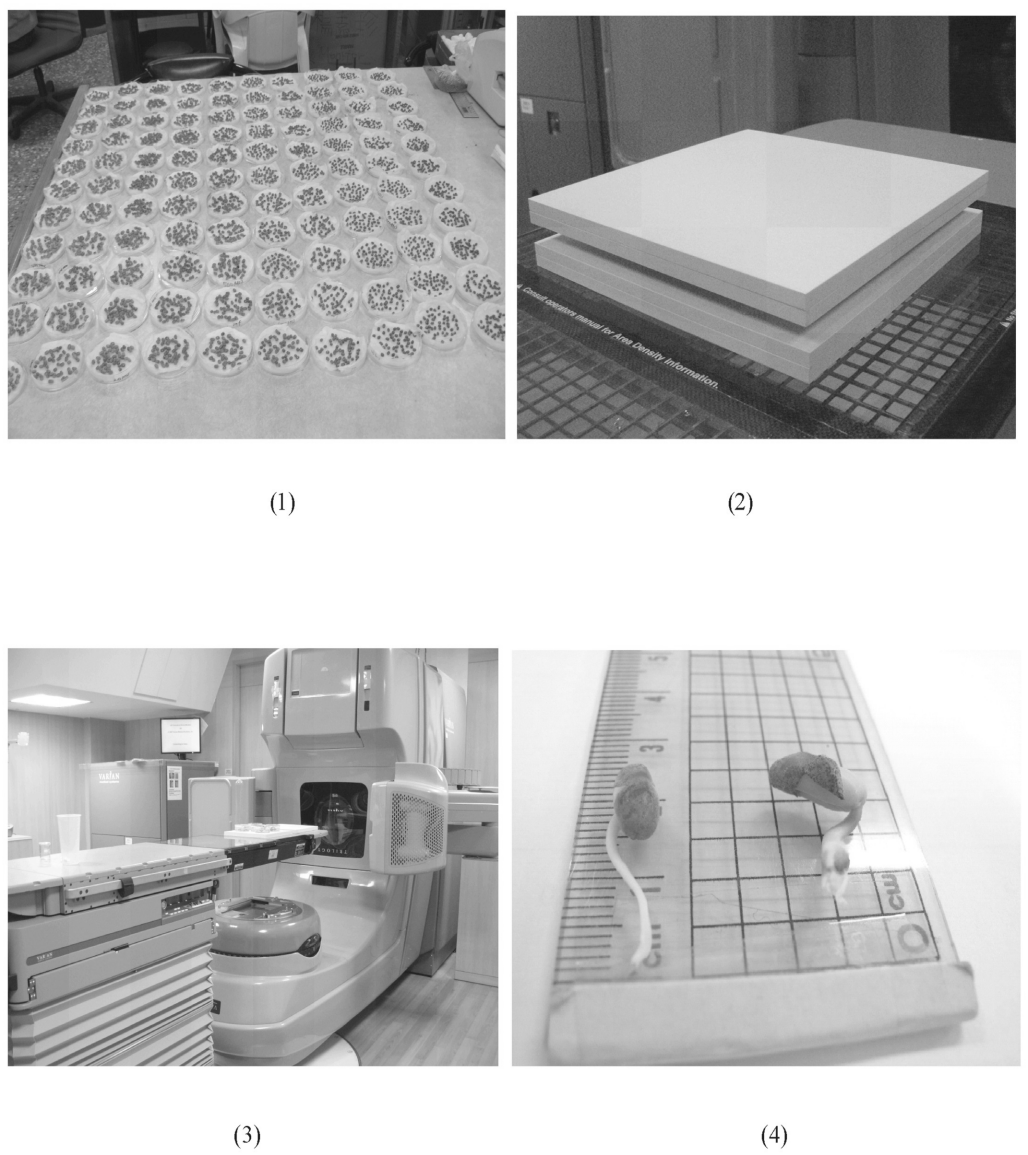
The real situation of mung bean inside the petri dishes (1) during watering, (2) between two 20 mm-thick solid polystyrene plates, (3) exposed to 6-MV LINAC with the head of LINAC placed bottom-down and the X-rays emitted from the bottom toward the top, and (4) during measurement with a ruler to within 1 mm.
